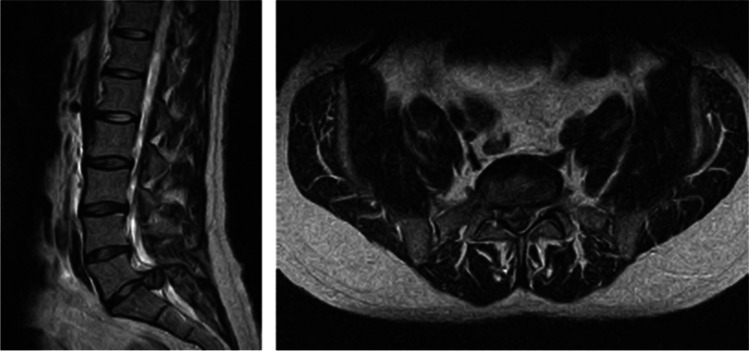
MRI scan example of a disc herniation causing cauda equina compression. The sagittal image on the left shows a large posterior disc herniation at L5/S1 level, and the axial scan on the right at the L5/S1 level shows that the disc herniation fills almost the entire spinal canal, and compresses the cauda equina
